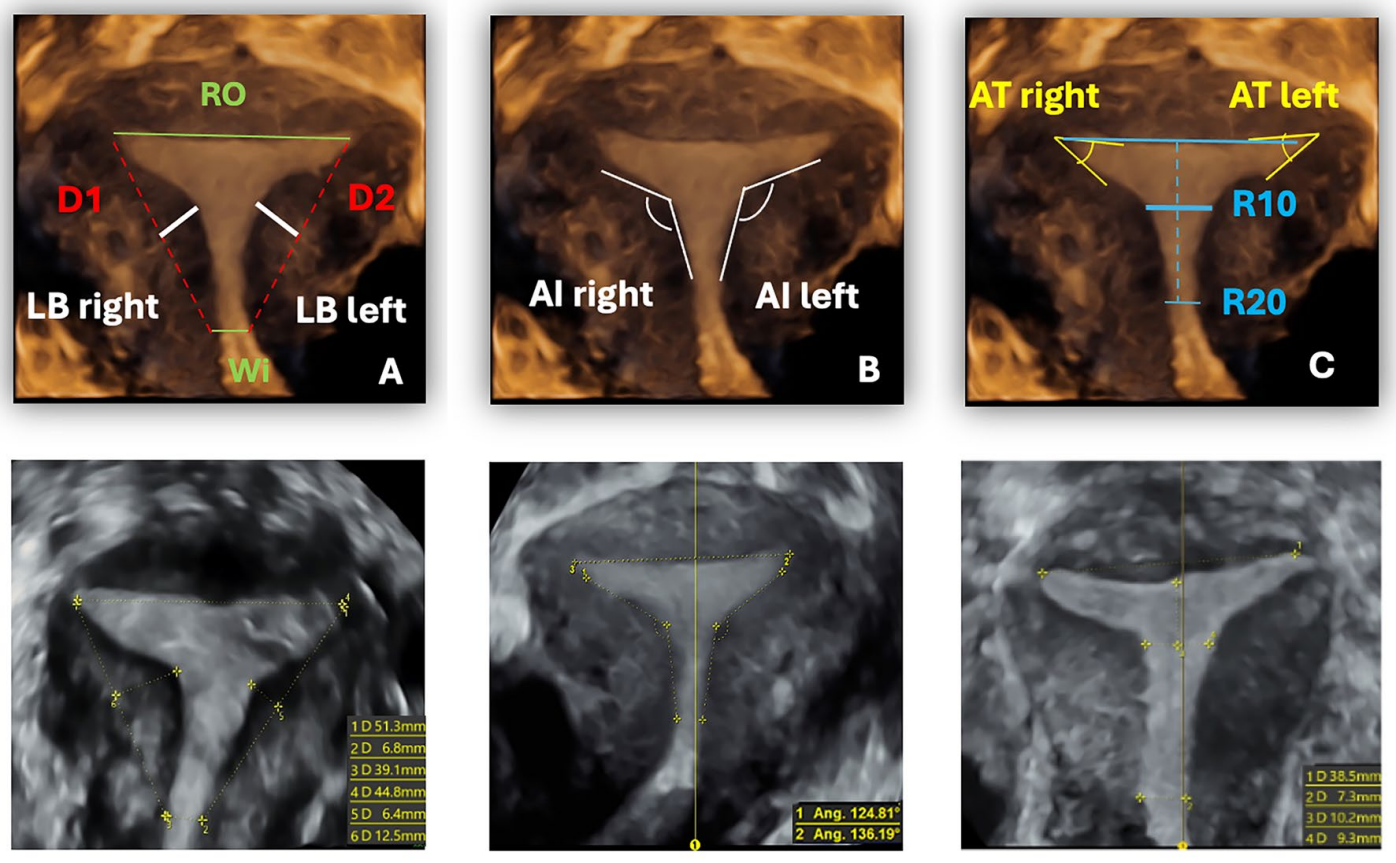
Uterine cavity measurements on transvaginal ultrasound 3D coronal view in T-shaped uteri according to the classifications currently in use. a—R0: fundal cavity width, the distance between the two internal tubal ostia.—Wi: corpus isthmic level cavity width, width of uterine cavity at the isthmic level or internal uterine orifice.—D1 right; D2 left: Distance between the tubal ostium and uterine isthmus/internal orifice.—LB right; LB left: lateral bulging, distance between D (right and left) and ipsilateral indentation angle AI. b —AI right; AI left: lateral indentation angle, the lateral angle between the corpus-isthmic cavity and the two fundal endometrial layers. c-AT right; AT left: T angle, the lateral angle between most lateral point of uterine cavity, contralateral most lateral point of uterine cavity and apex of ipsilateral lateral indentation.—R10: length of the intracavitary line parallel to the interostial line at 10 mm from it.—R20: length of the intracavitary line parallel to the interostial line at 20 mm from it
